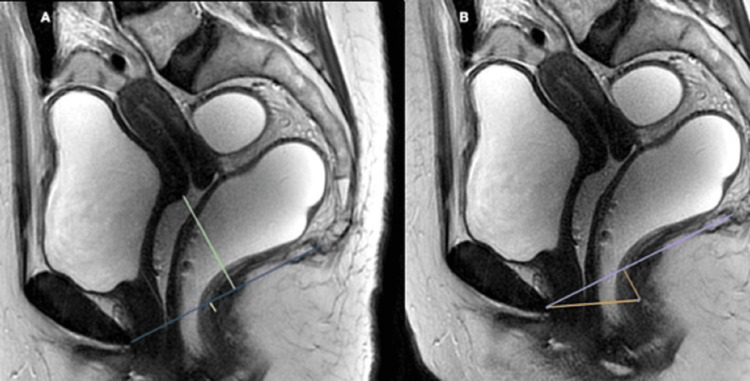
Sagittal T2WI (A) depicting the pubococcygeal line drawn from the inferior aspect of the pubic symphysis to the last coccygeal joint. The most posterior and inferior aspects of the bladder base, the anterior cervical lip, and the posterior wall of the anorectal junction are taken as the reference points in the anterior, middle, and posterior compartments, respectively. The sagittal T2WI (B) depicting the H line (yellow line) and the M line (pink line). T2WI: T2-weighted image.
